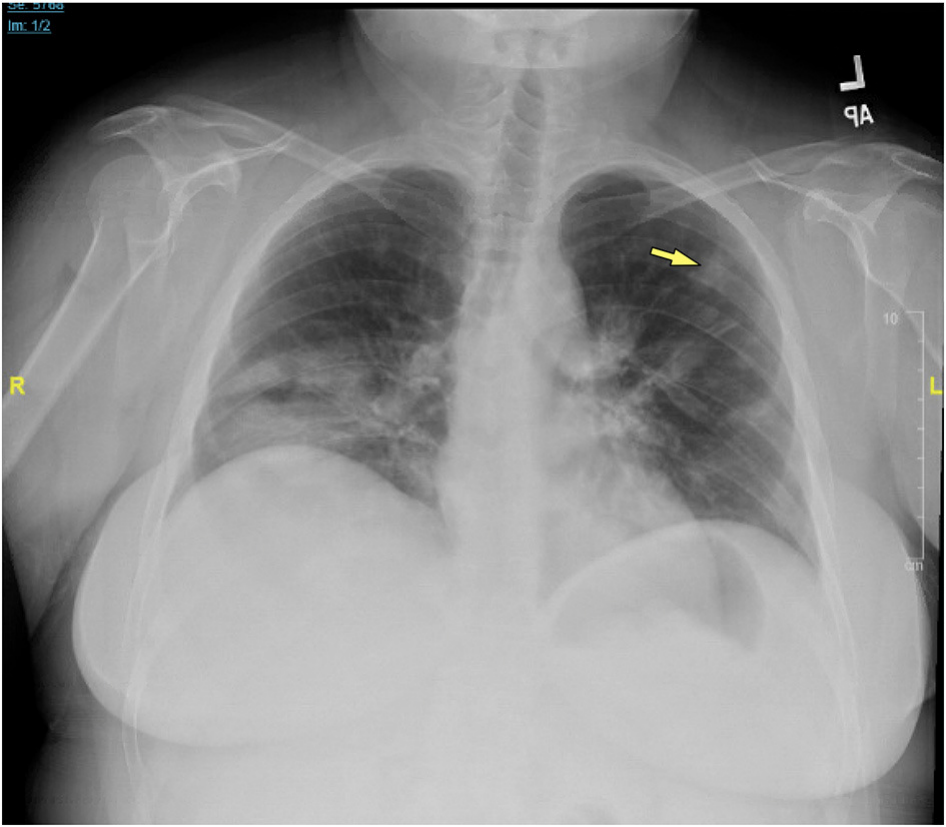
Chest x-ray of CVID/ILD patient demonstrating presence of 1.6 cm lesion in the left upper lobe (yellow arrow), as well as patchy densities in the mid to lower lung fields.
